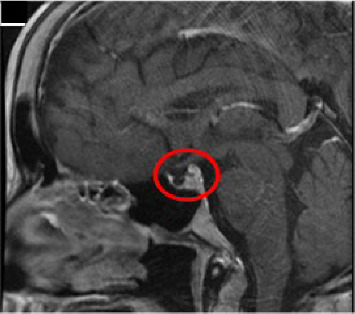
Imaging of the pituitary gland in Sheehan's syndrome: MRI showing empty sella turcica. Magnetic resonance imaging (MRI) is considered the gold standard for diagnosing Sheehan's syndrome. The pituitary gland resides within the sella turcica, a depression in the sphenoid bone. In cases of pituitary necrosis, this space often appears partially or completely empty on imaging. Studies suggest that around 70% of Sheehan's syndrome cases demonstrate a fully empty sella turcica. While MRI provides the most detailed visualization, computed tomography (CT) scans can also aid in the diagnostic process (Figure 1).
